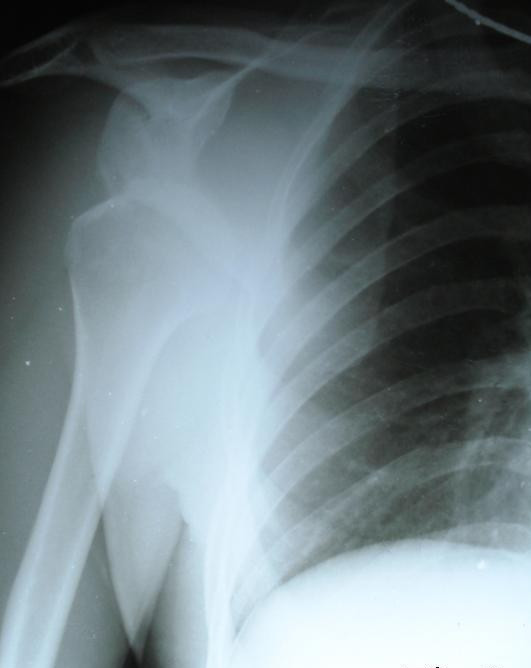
Pre-reduction radiograph showing subglenoid inferior dislocation with humerus parallel to the chest wall.
